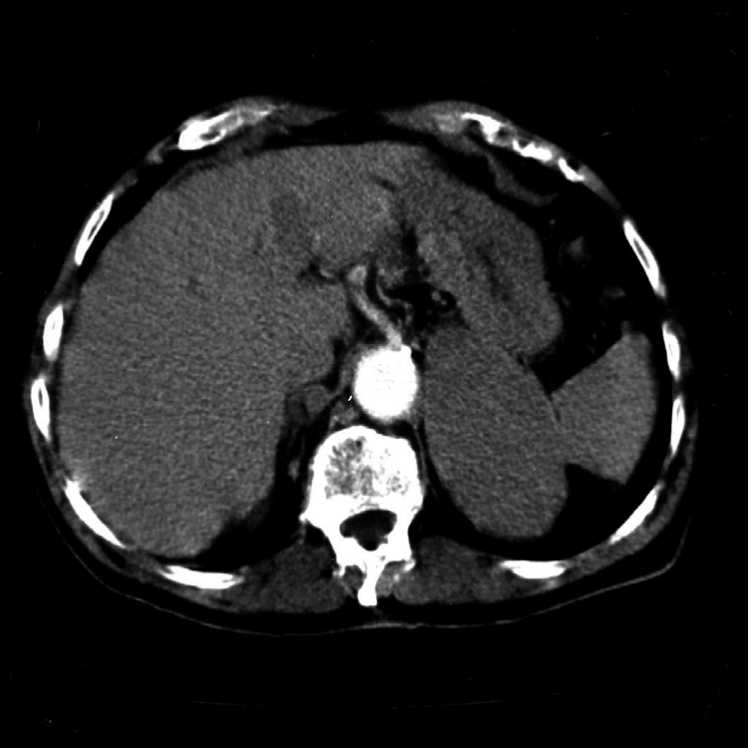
CT scan showing left adrenal gland tumor 10 cm in diameter, having homogenous intensity. No liver lesion is visualized.
